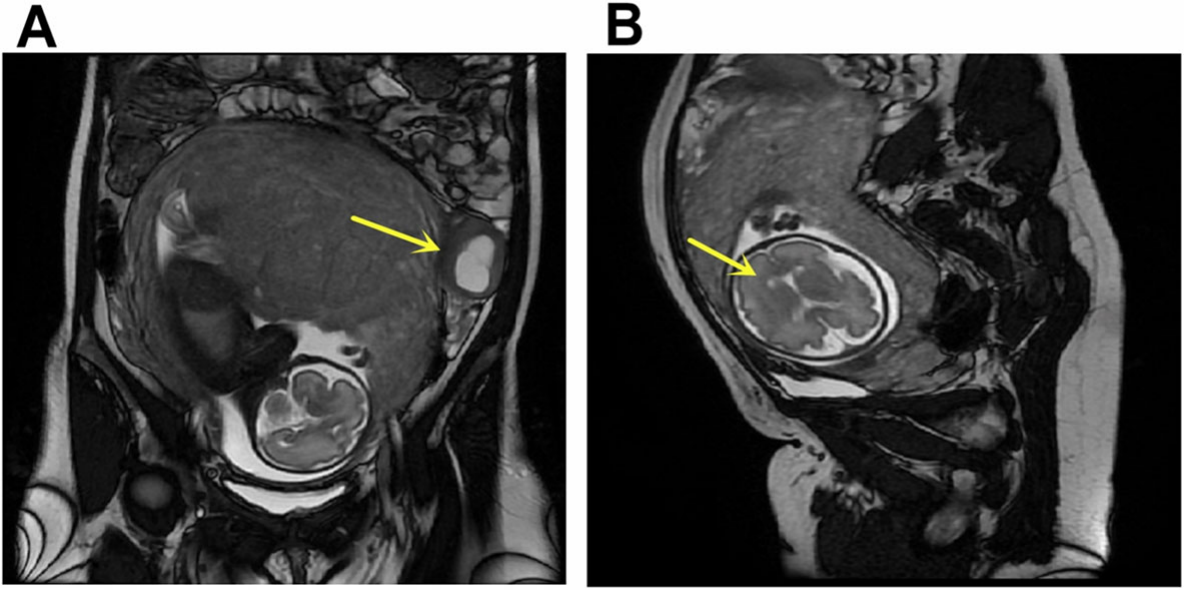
MRI shows a large mass on left ovary(a) and bilateral subependymal cysts(b)
